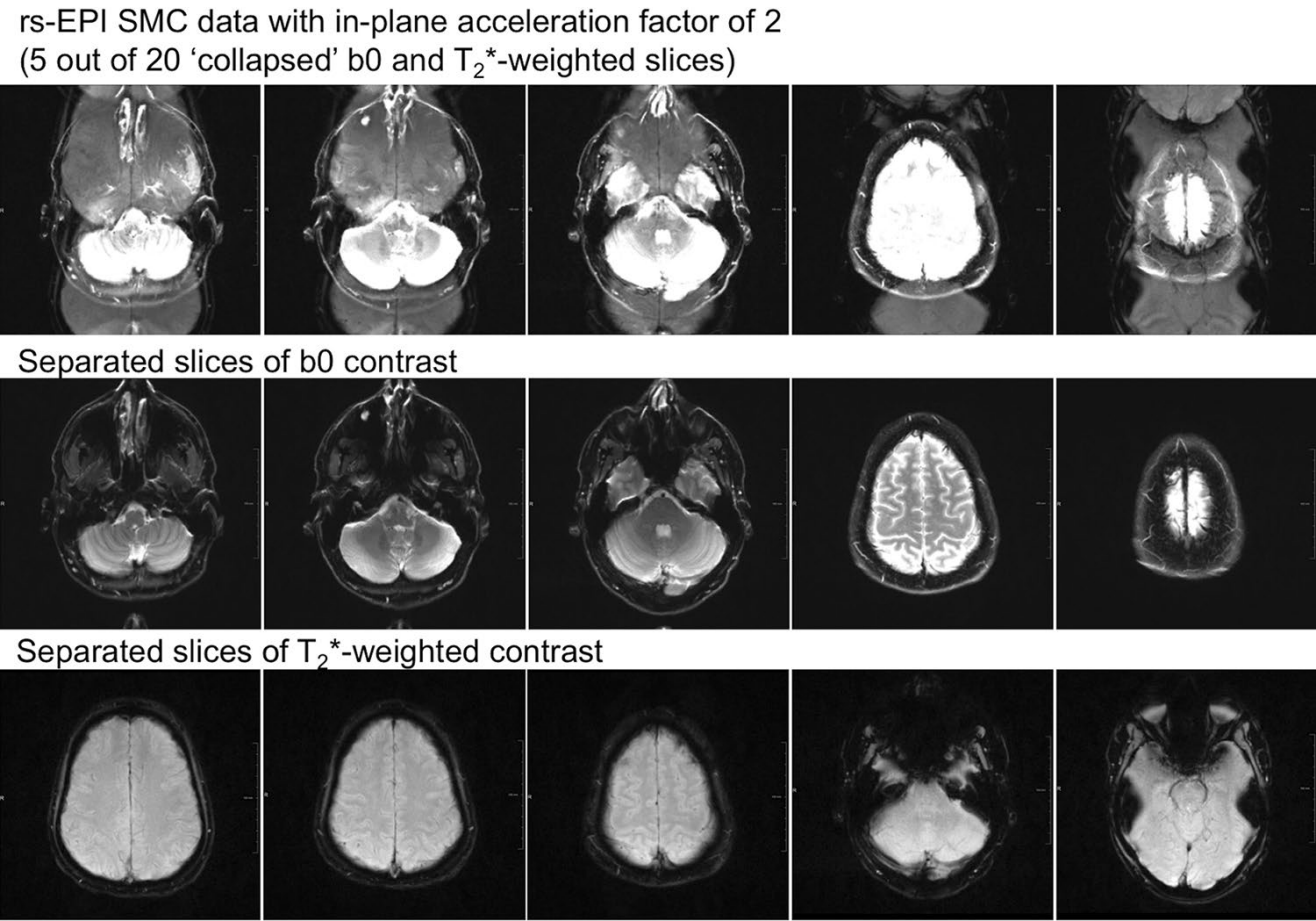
Data from five out of 20 measured SMC slices. The first row shows the collapsed SMC data with an in-plane acceleration factor of 2; The slices from the DW acquisition (with *b* = 0 s/mm^2^) are at the center of the FOV while the T_2_*W slices are shifted by FOV/2 in the anterior–posterior direction. The middle row shows the DWI data and the bottom row shows the T_2_*W data
